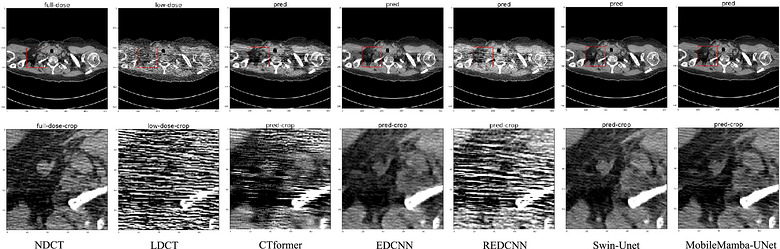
A detailed visual comparison on an abdominal CT slice randomly selected from the test set of the Mayo‐2020, displayed within the intensity window of [−1000,2000] HU.
